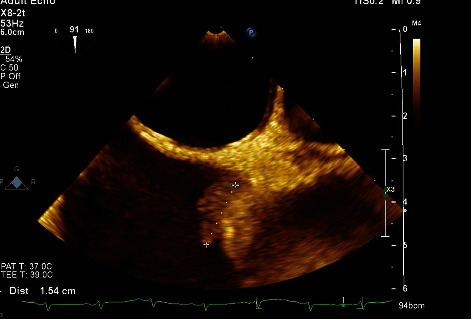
Transesophageal echocardiogram showing a mobile lesion at the junction of the SVC and right atrium. The lesion is irregular and measures about 1.7 cm in greatest dimension.
